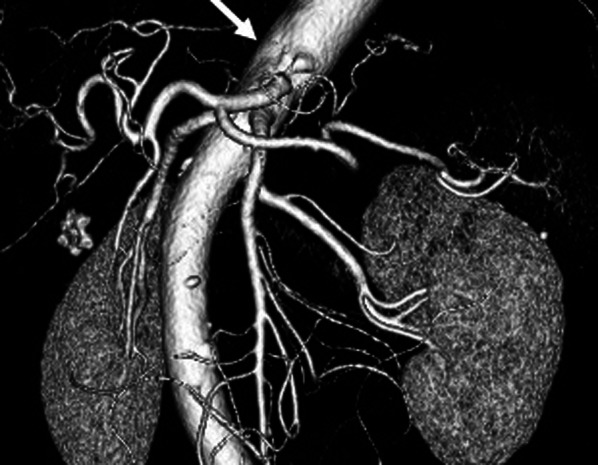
3-D CT angiography showed stenosis of the celiac artery due to MALS (allowed)
